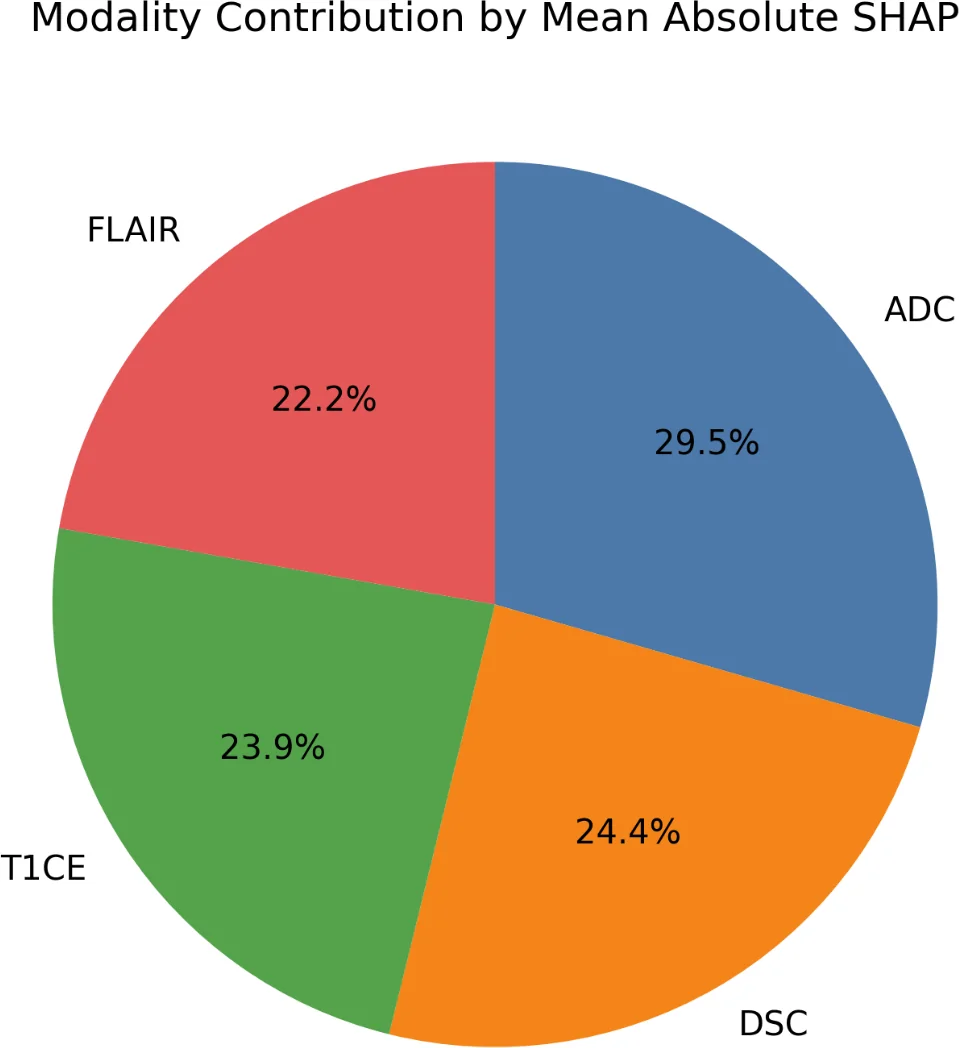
Modality contribution by mean absolute SHAP. Mean absolute SHAP values were aggregated by imaging modality. ADC contributed 29.5% of total attribution, followed by DSC at 24.4%, T1CE at 23.9%, and FLAIR at 22.2%, indicating balanced multimodal contribution across diffusion, perfusion, postcontrast, and FLAIR-derived radiomic information.
